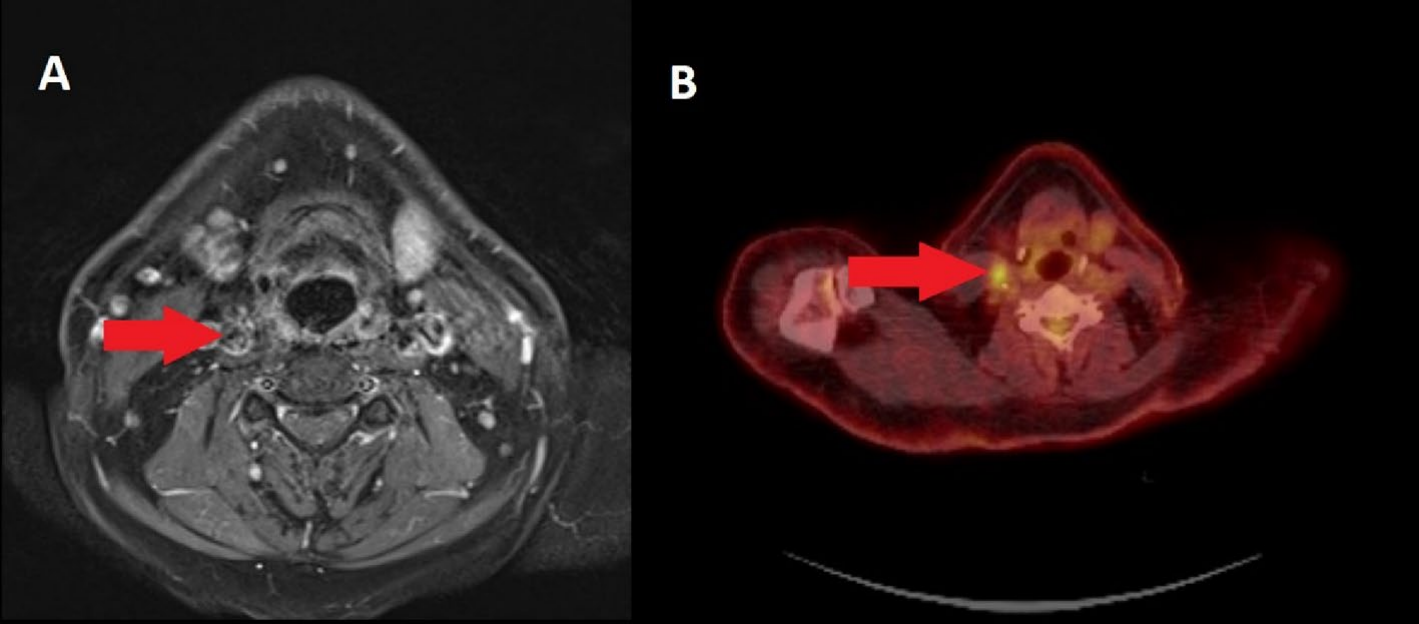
PET/CT showed a false positive result in a negative neck. The area of FDG-uptake (B) and the related node in the MRI (A) are marked by red arrows. The time from MRI to surgery was 36 days, from PET/CT to surgery 29 days in this case. Inflammation is discussed as a major reason for false positive results in PET/CT.
